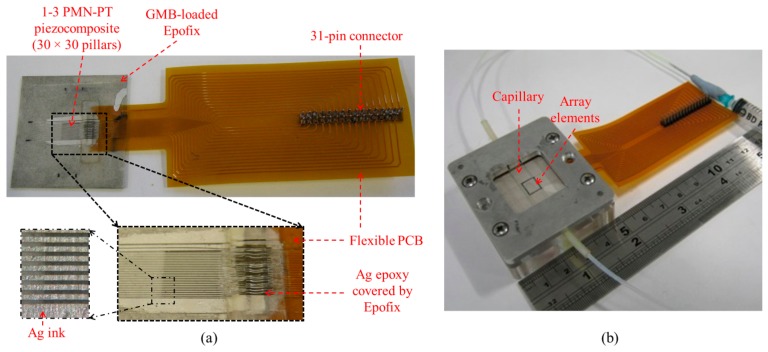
(a) 1-D 30-element ultrasonic array; and (b) complete device in a housing with a capillary channel.
